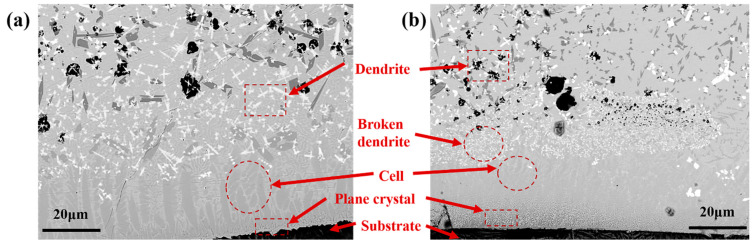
Microstructures of the junction between the cladding layer bottom and the substrate (a) without ultrasonic vibrations and (b) with ultrasonic vibrations (UP = 800 W, F = 32 kHz).
